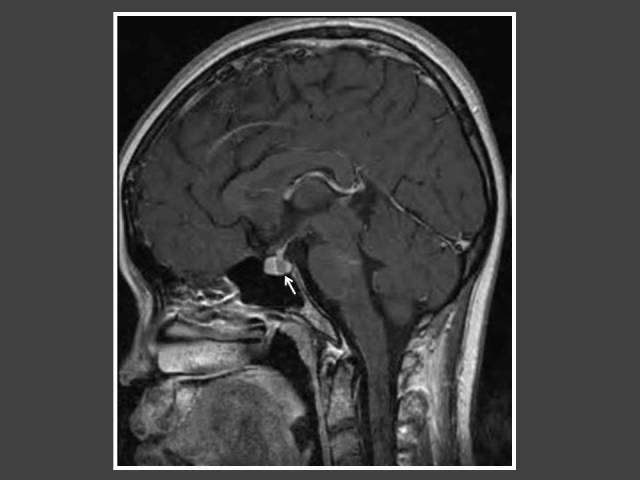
A girl with global developmental delay who presented with bilateral breast enlargement. T1WI Sagittal MRI of the brain (post-gadolinium dynamic protocol) shows an intrasellar lesion representing pituitary microadenoma (arrow).
